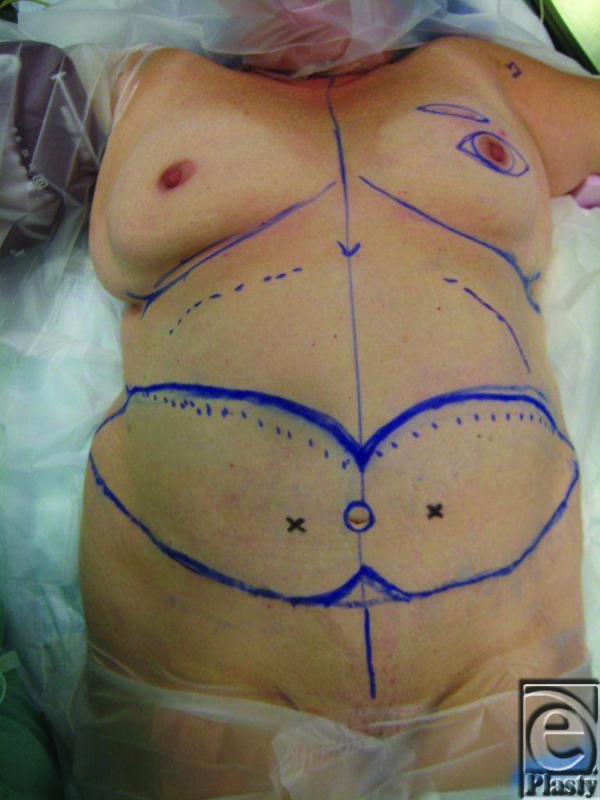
Initial flap design with markings of the right and left perforators found on Doppler auscultation.
